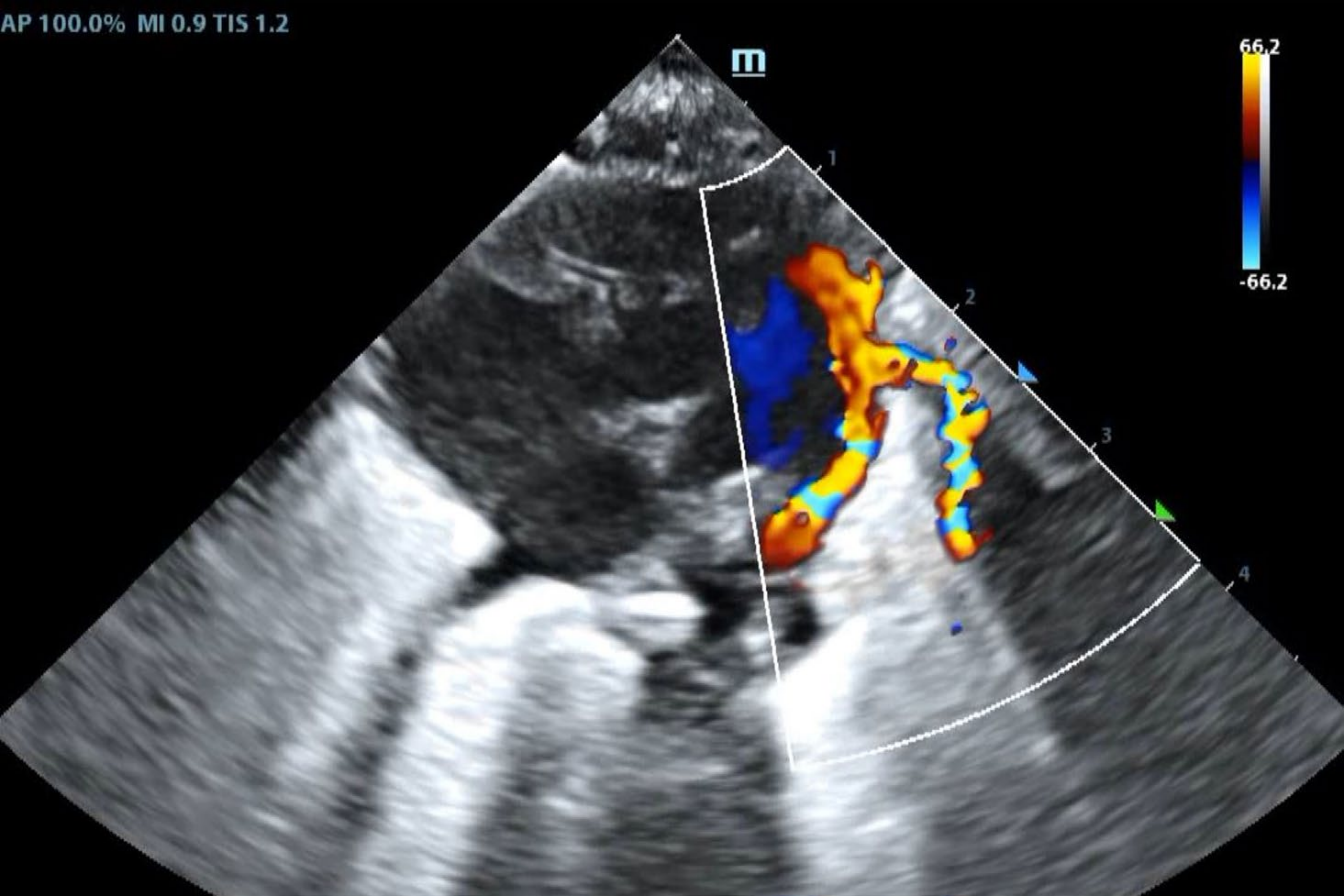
Echocardiography performed 1 week after birth revealed a patent ductus arteriosus, which was managed conservatively with no surgical or pharmacological management.
